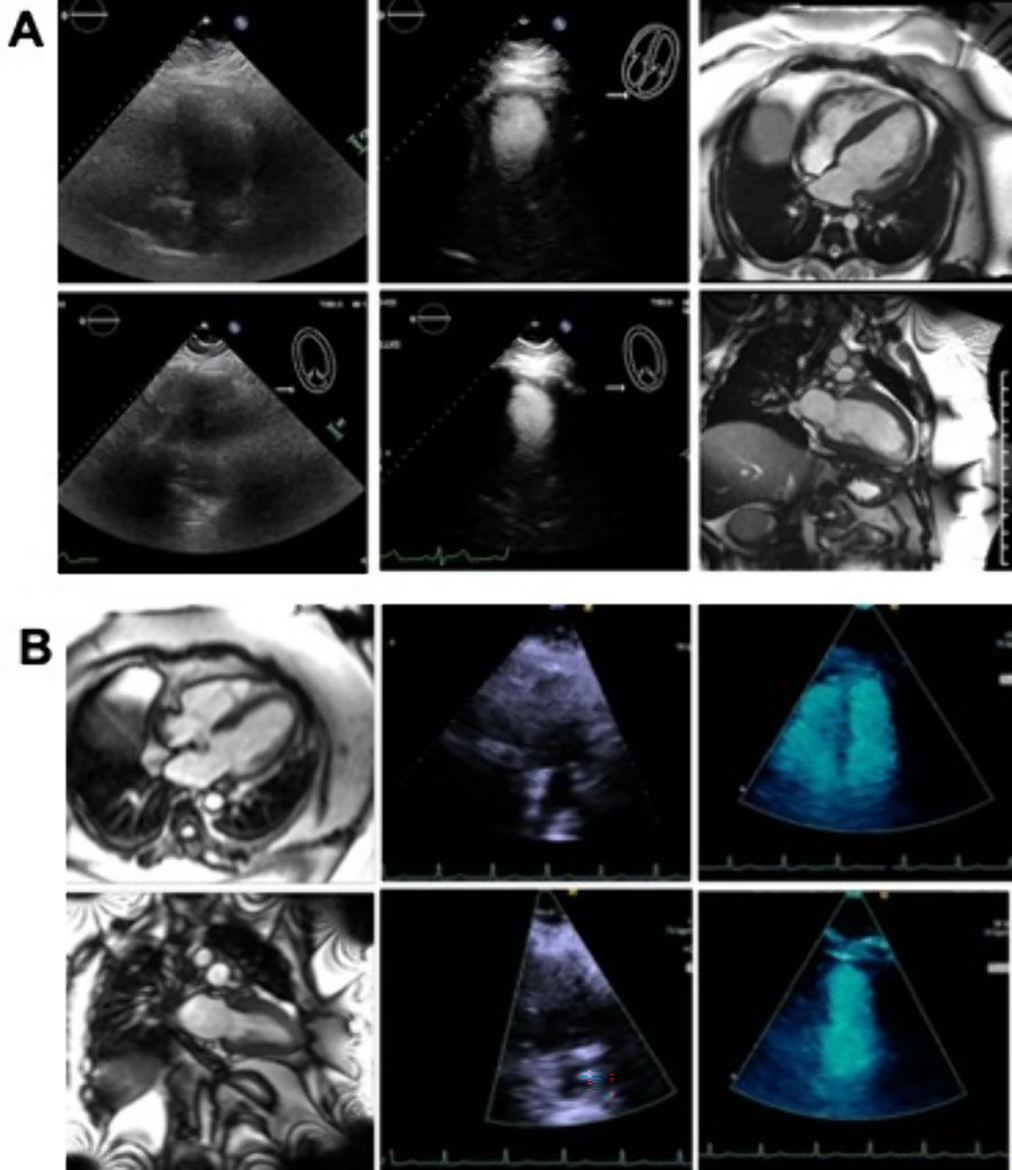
A Example of low overall echocardiography image quality score. Top (left to right) – 4 chamber TTE views without, with microbubble contrast, MRI steady state free precession cine. Bottom: 2 chamber TTE views without, with microbubble contrast, MRI steady state free precession cine. Overall image quality score: TTE – 21; MRI – 91. *TTE* transthoracic echocardiography, *MRI* magnetic resonance imaging. BMI 49.2. B Example of low overall cardiac magnetic resonance image quality score. Top (left to right) – 4 chamber MRI steady state free precession cine, TTE view without, with microbubble contrast. Bottom: 2 chamber MRI steady state free precession cine, TTE view without, with microbubble contrast. Overall image quality score: MRI – 71; TTE – 43.5. *TTE* transthoracic echocardiography, *MRI *magnetic resonance imaging. BMI = 56.5
